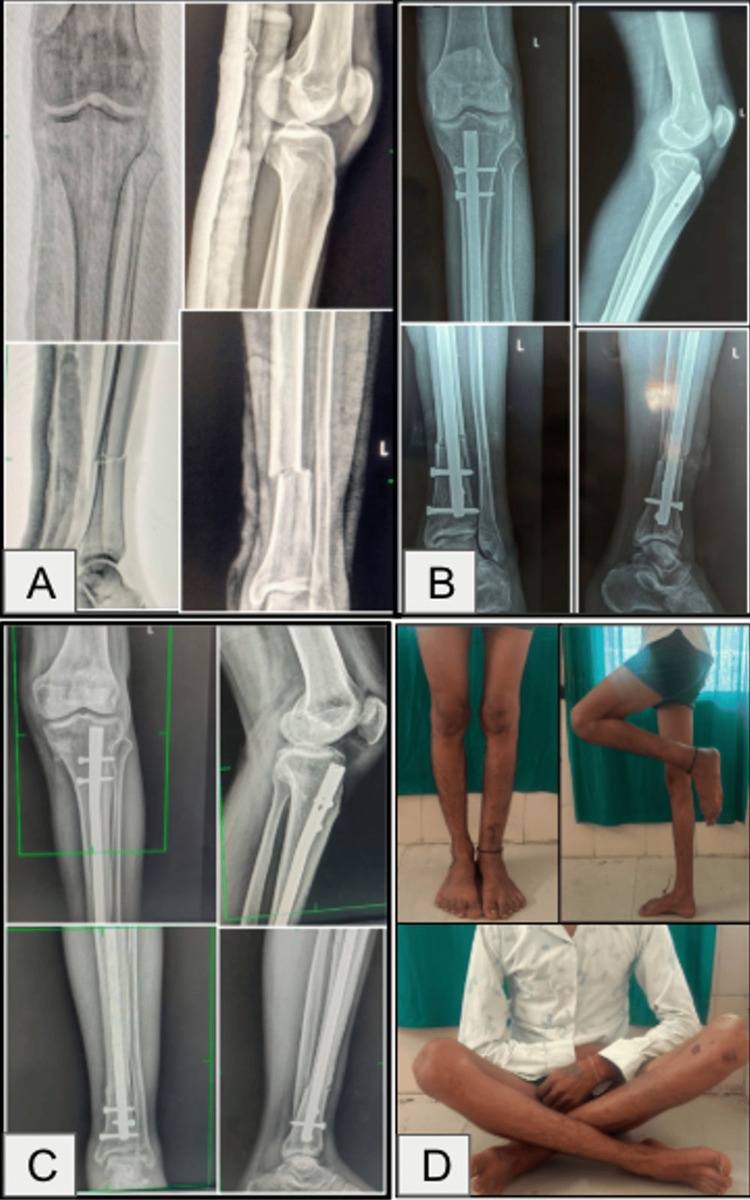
Case 2 of infrapatellar nailing. (A) Preoperative X-ray. (B) Immediate postoperative X-rays. (C) Three months postoperative X-rays. (D) Clinical assessment and knee range of motion at three months.
